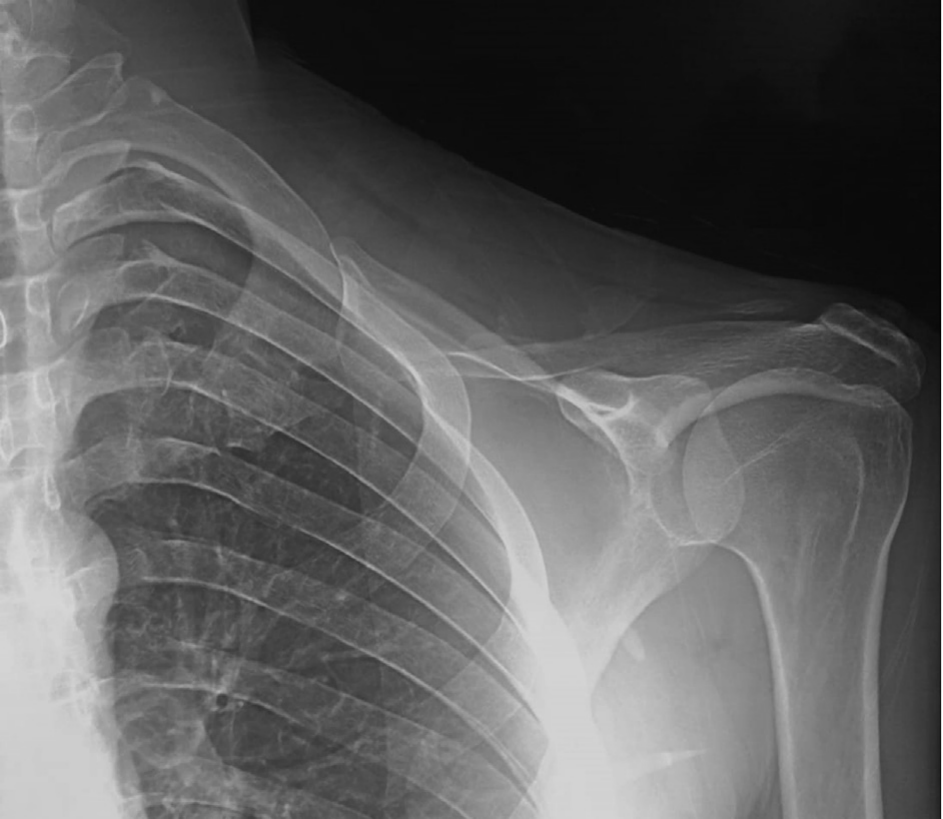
Postoperative radiographs of the shoulder girdle after total claviculectomy.
